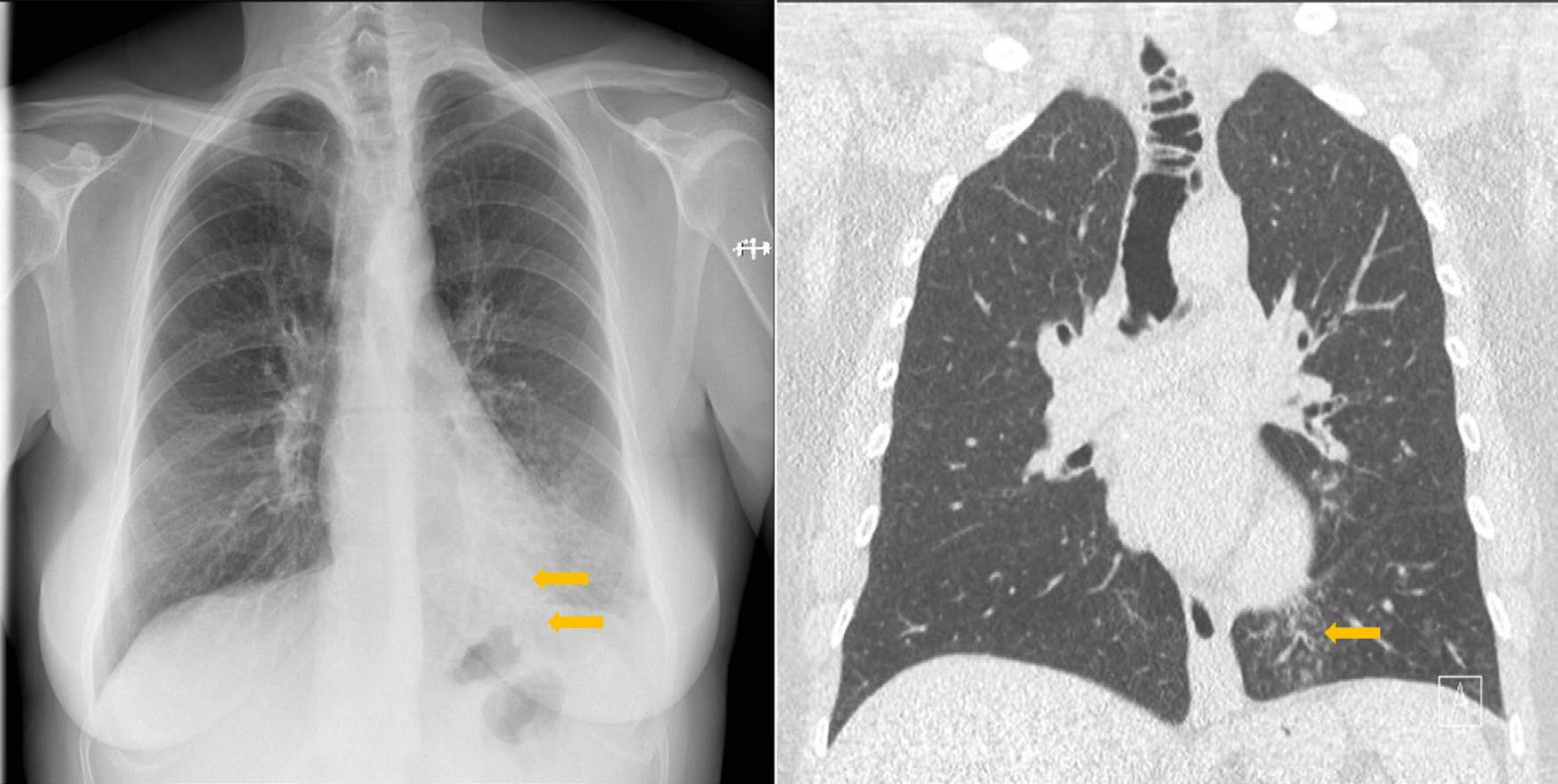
Tracheal diverticulosis and left bronchiolitis; frontal CXR and CT comparison.
